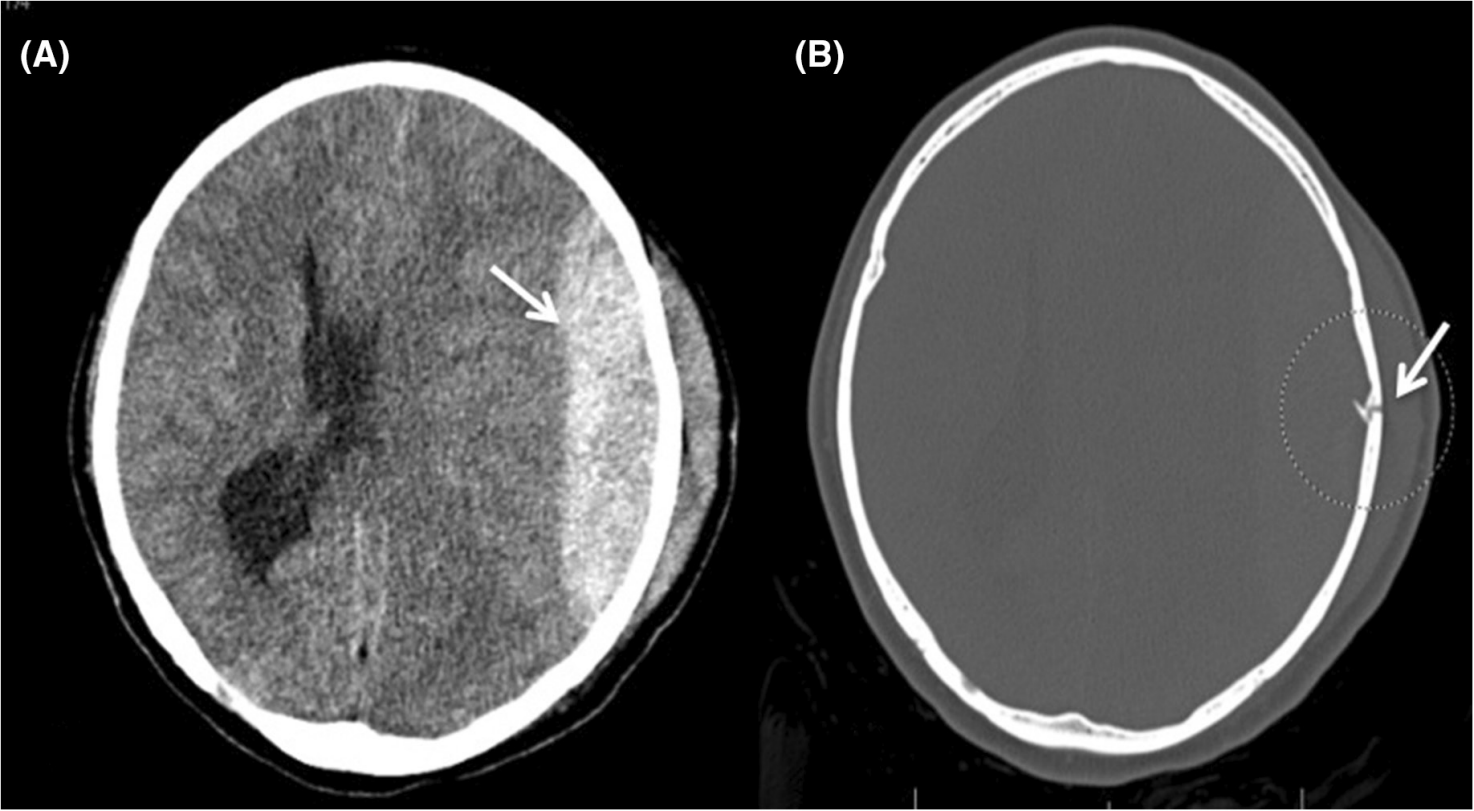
(A) Postoperative skull computed tomography showing large acute epidural hematoma. (B) Computed tomography with bone window showing fracture related to head fixating pin.
